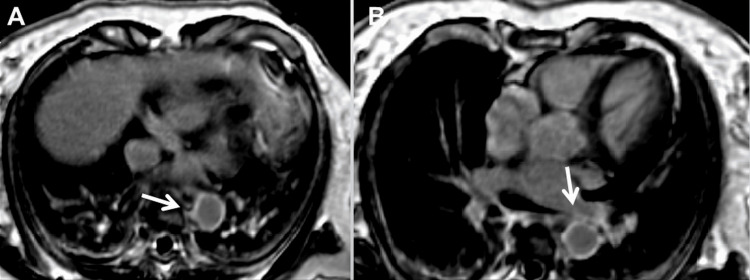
Computed tomography of the thorax showing “coating” of the thoracic aorta (A, B), suggestive of ECD.
